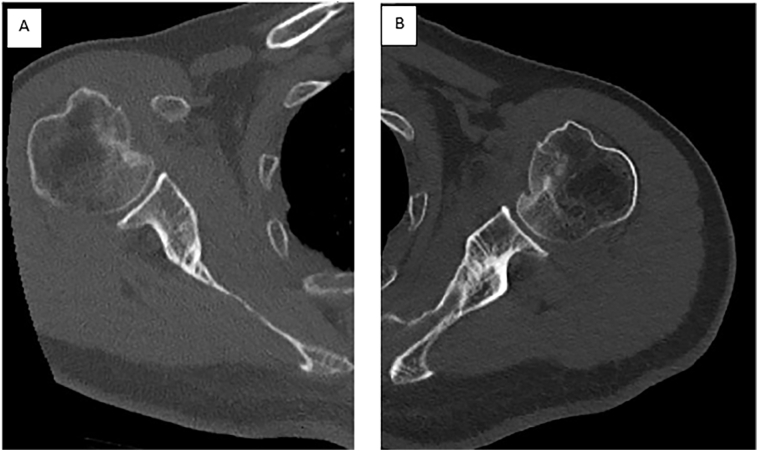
CT of both sides confirming the dislocation and measurements of Reverse Hill Sach lesion A: Right shoulder, B: left shoulder.
